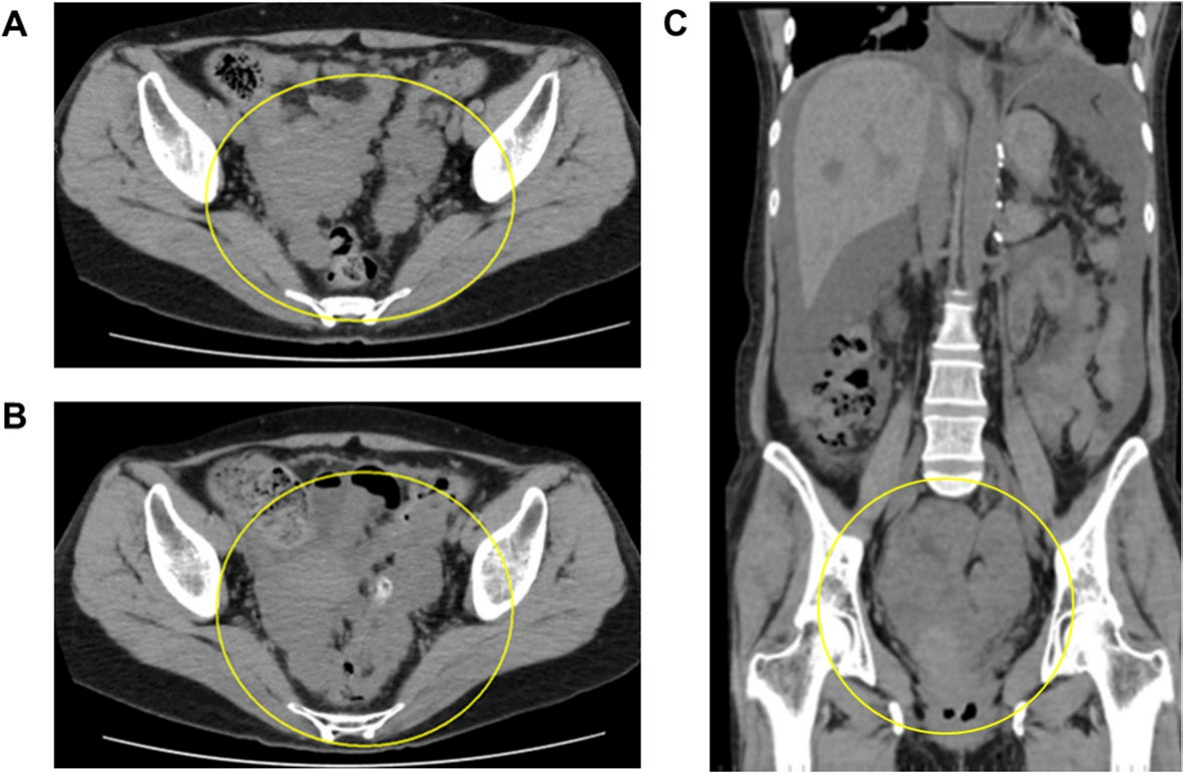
CT images. A One month before admission. B At the time of admission, CT showed that the progression of disseminated lesions in the pelvis was observed. C Coronal view at the time of admission: massive ascites and disseminated metastasis of pheochromocytoma surrounding the uterus
